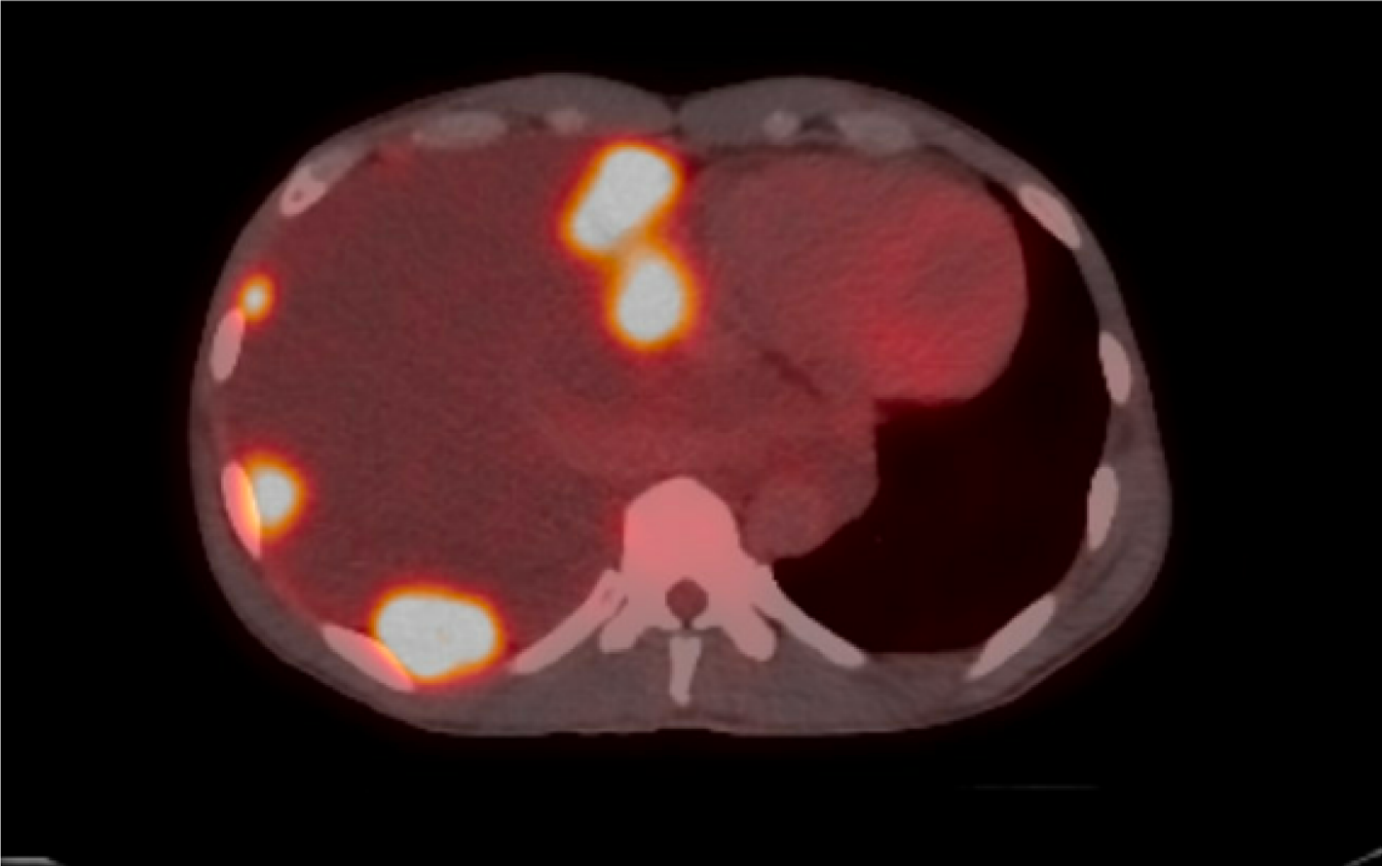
Positron emission tomography/computed tomography (PET/CT) showed innumerable FDG‐avid right pleural‐based lesions with a maximum standardized uptake value (SUV) of 14.3, as well as fluorodeoxyglucose (FDG)‐avid right hilar and bilateral mediastinal adenopathy.
